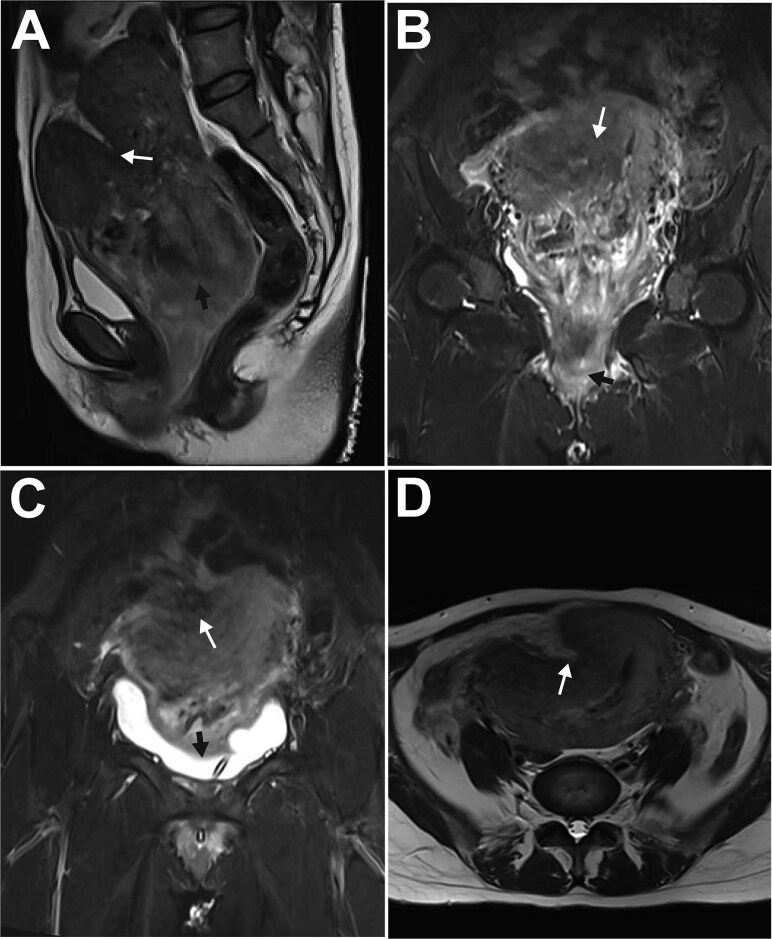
MRI features of subacute uterine inversion secondary to a large uterine myoma. A: Paramedian sagittal T2-weighted turbo spin-echo image showed a lobulated fundal depression (white arrow) and the underlying uterine myoma (black arrow); B: Paramedian sagittal T2-weighted turbo inversion recovery magnitude (TIRM) image demonstrated the myoma prolapsing from the uterine cavity into the vagina (white arrow), with a portion extending through the introitus (black arrow); C: Coronal T1-weighted image revealed the myometrial indentation (white arrow) and the compressed urinary bladder (black arrow); D: Axial T2-weighted turbo spin-echo image showed the myometrial depression (white arrow) on the right anterofundal wall.
